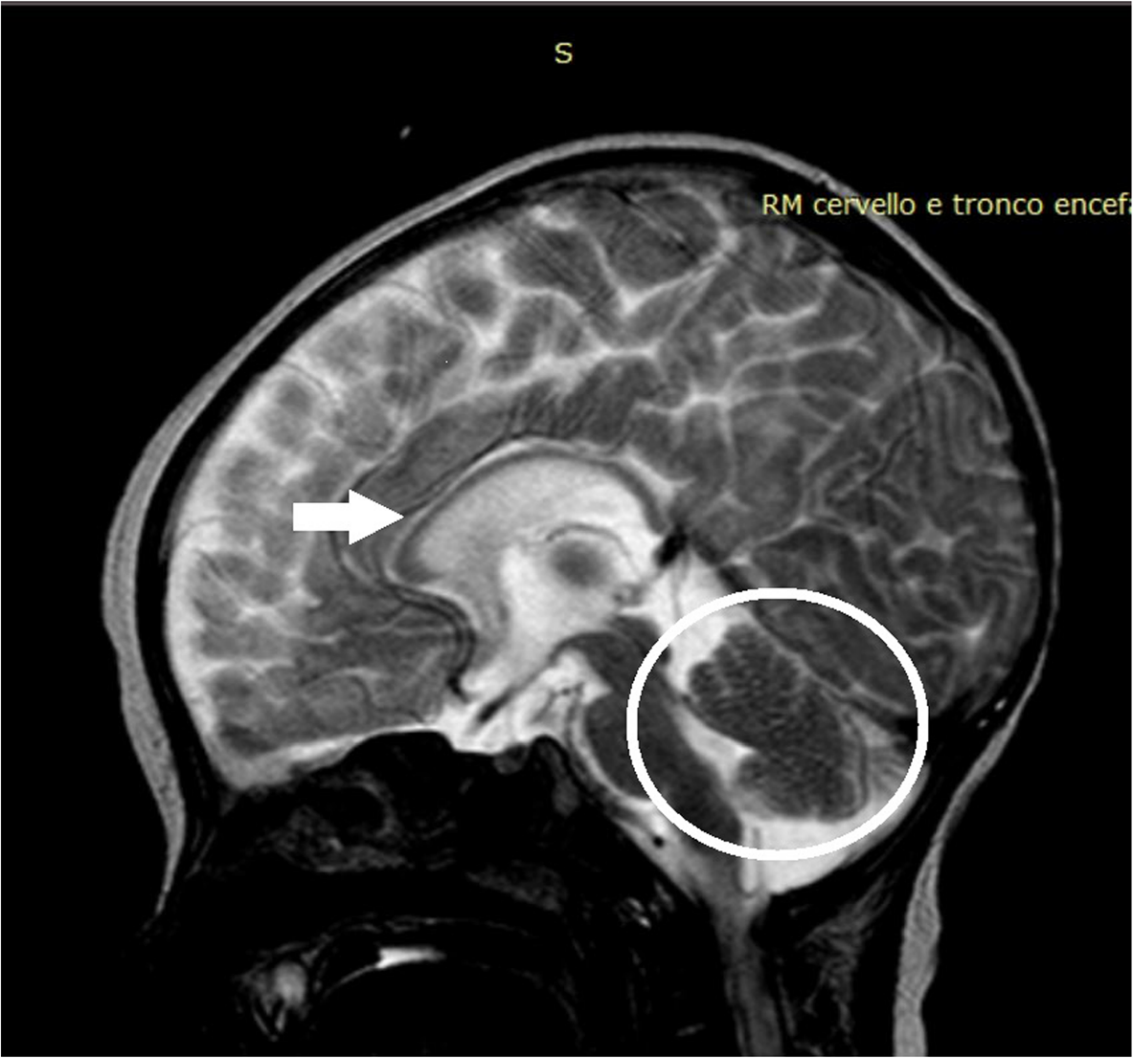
Brain MRI T2 sagittal image: mild diffuse cerebral atrophy, hypoplasia of the corpus callosum (white arrow), cerebellar vermis hypoplasia (white circle)
